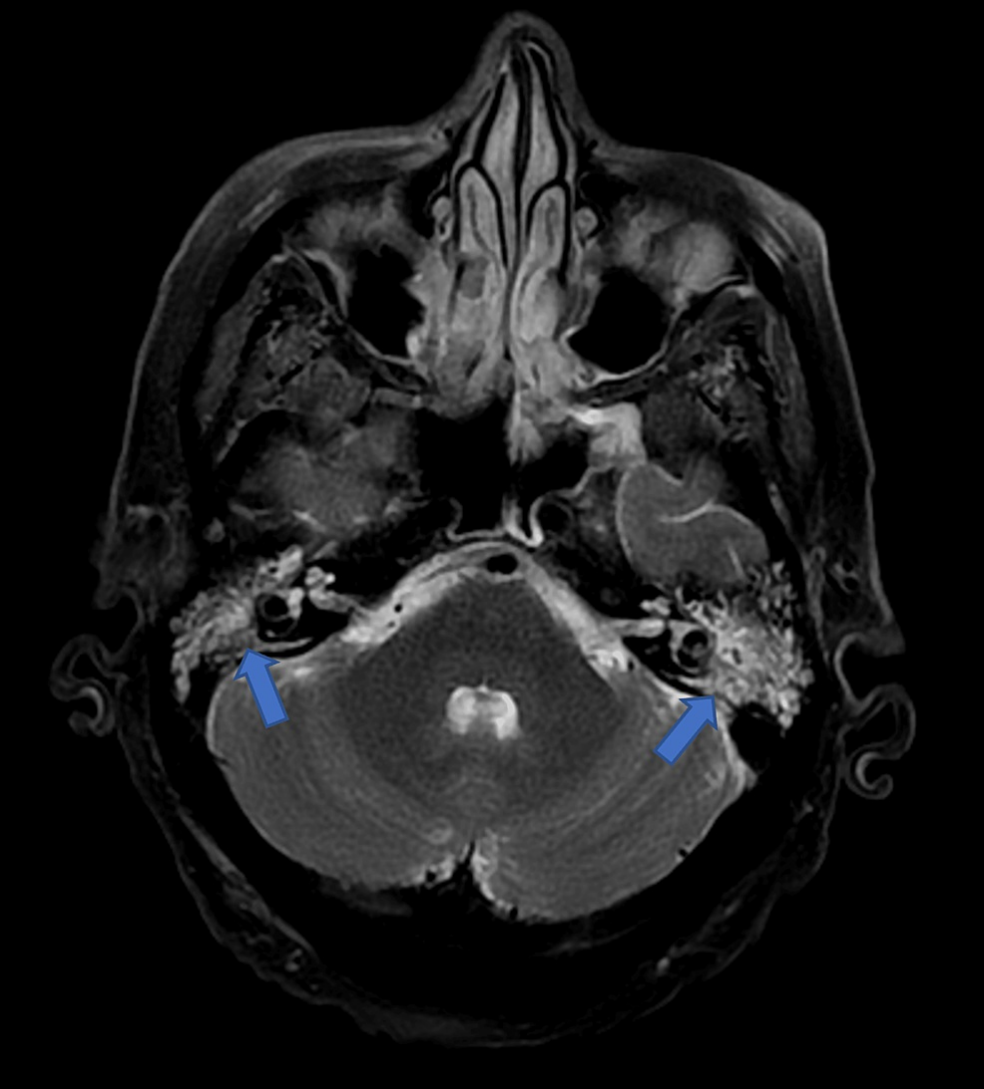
T2-weighted magnetic resonance image of the brain demonstrating bilateral erosive mastoiditis (blue arrows).
